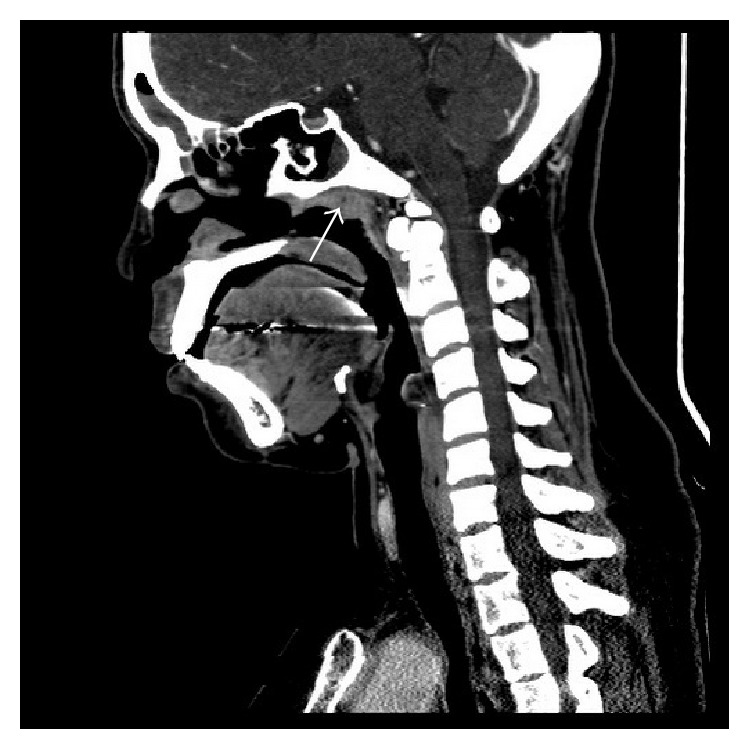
A 32-year-old woman, sagittal contrast-enhanced CT shows small central necrosis area in the nasopharyngeal lesion.
